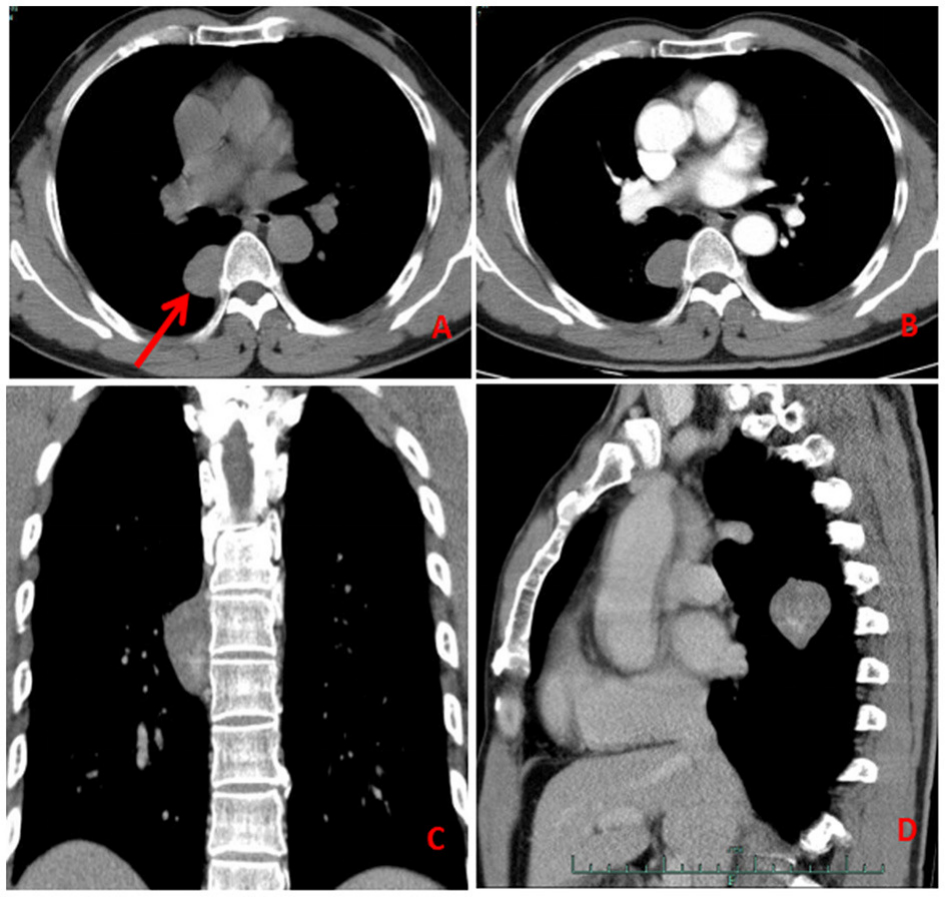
Computed tomography (A: axial, C: coronal, D: sagittal) of the chest showed a mass in the posterior mediastinum (red arrow). The enhancement scan (B) was uneven and slightly enhanced.
